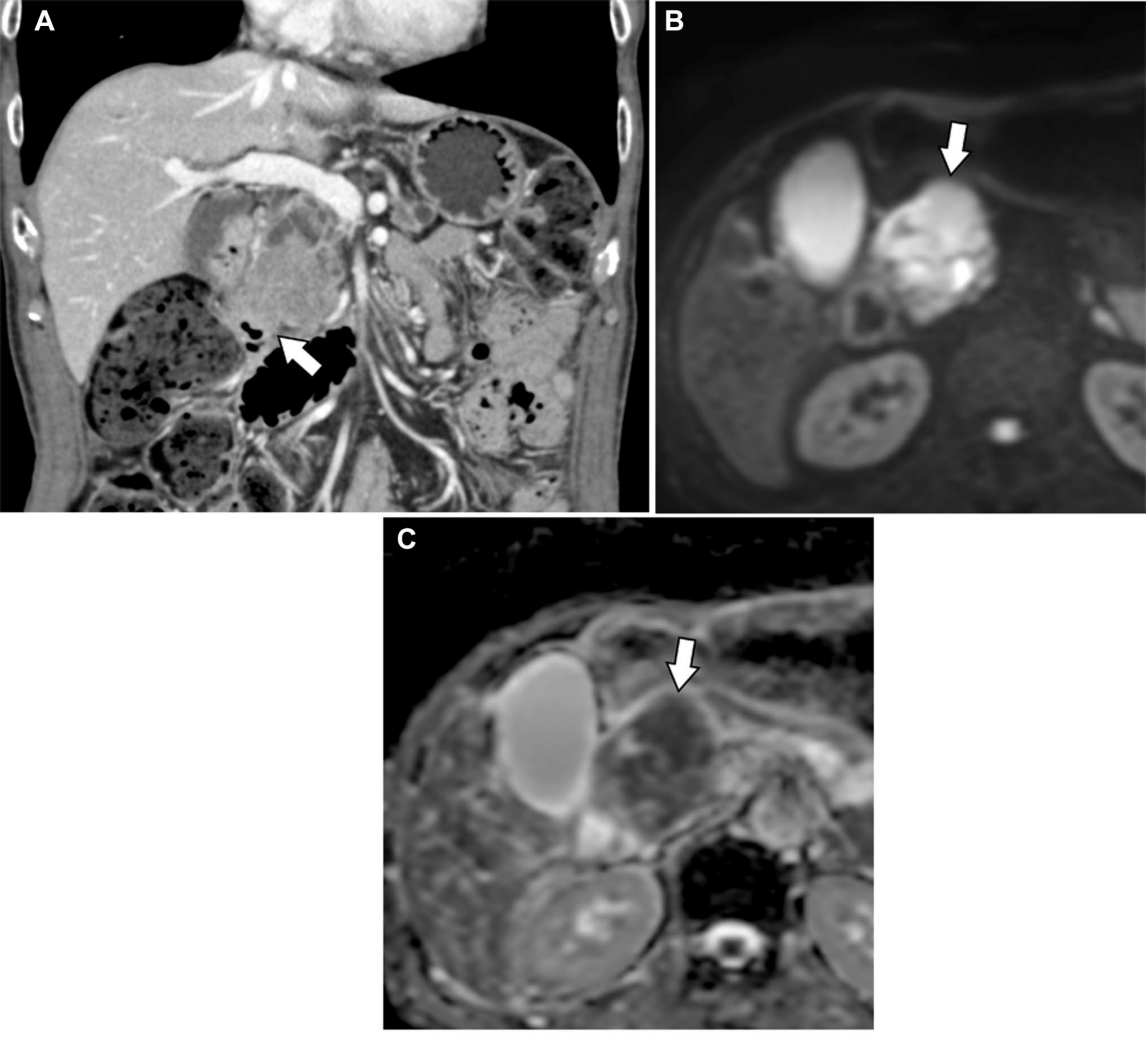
Case of a 72-year-old man with a chief complaint of weight loss shows a mass in the head of the pancreas (Case 15). Coronal contrast-enhanced computed tomography reveals a pancreatic head mass invading the duodenum (a: arrow). On diffusion-weighted image, the tumor shows high intensity (b: arrow), and the apparent diffusion coefficient value is low (1.02 × 10^–3^ mm^2^/s) (c: arrow). Pathological examination reveals the tumor has invaded the proper muscular layer of the duodenum and accessory papilla.
